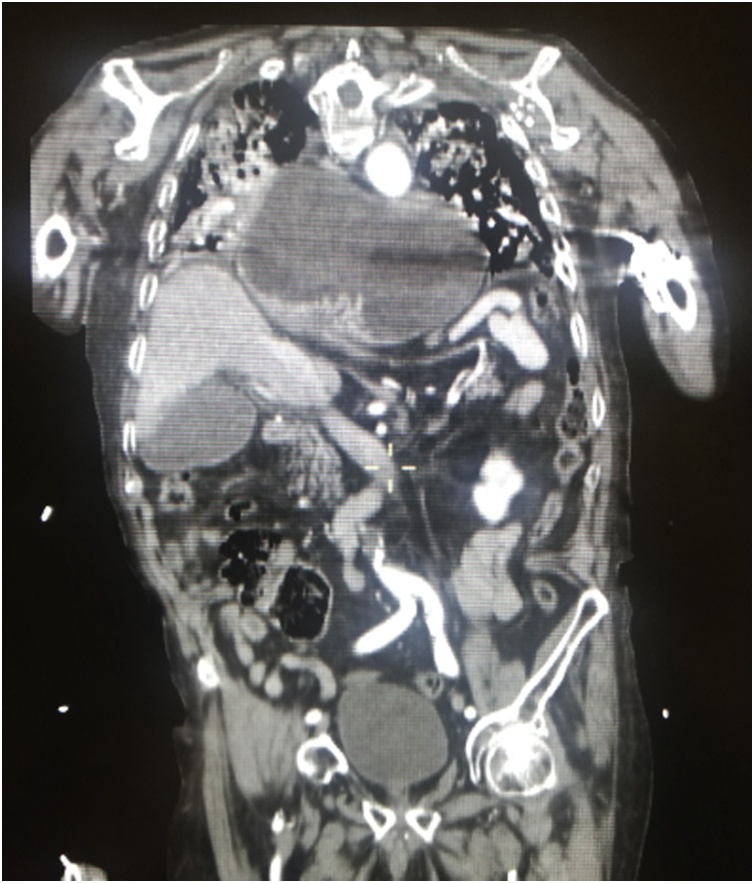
Abdominal CT. Complete intramediastinal volvulus.
